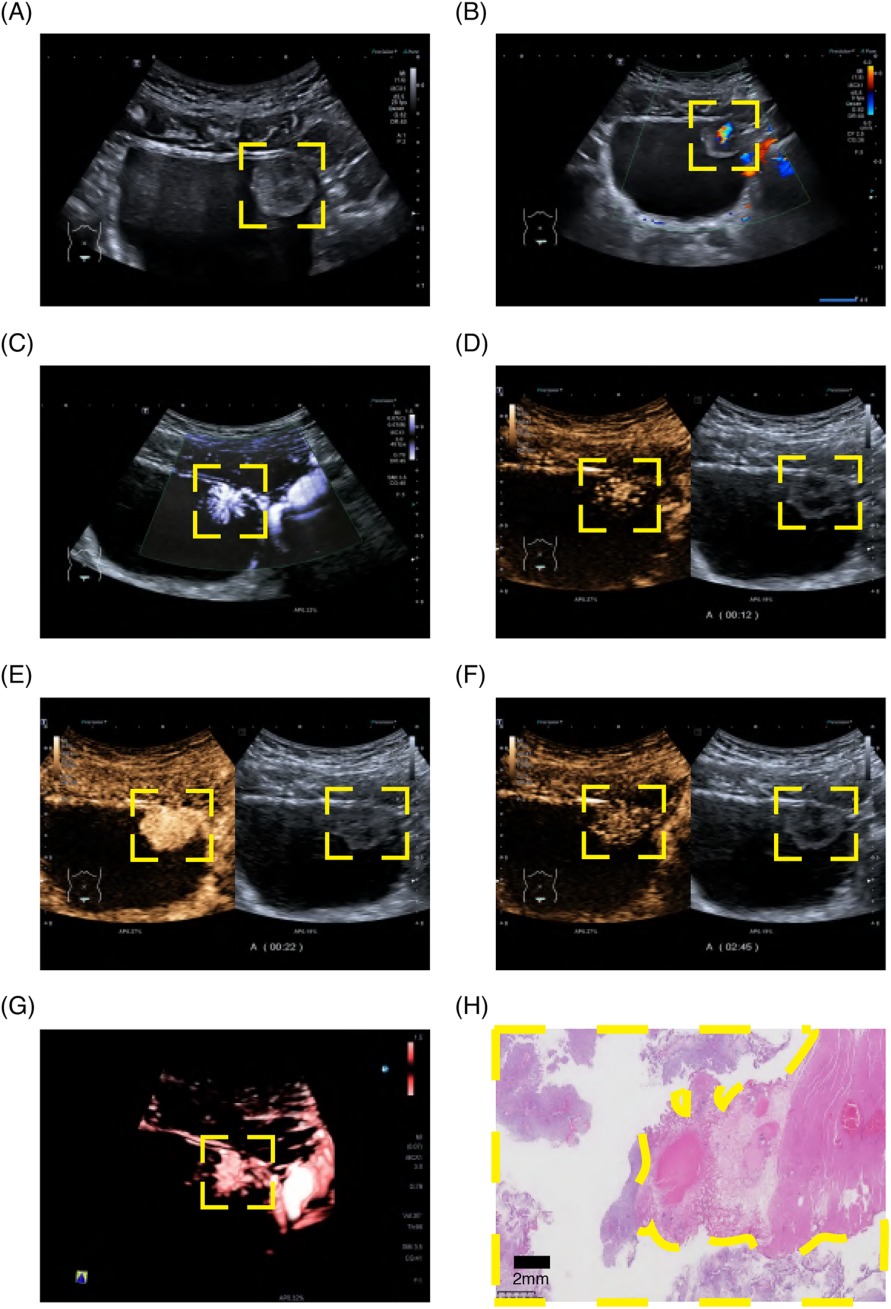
Ultrasound characteristics and pathology status of nonmuscle invasive bladder cancer patients and pathology status. (A) Gray‐scale ultrasound; (B) color Doppler flow imaging; (C) contrast harmonic imaging + superb micro vascular imaging; (D) contrast harmonic imaging in 12 s just before the lesion began to strengthen; (E) contrast harmonic imaging in 22 s almost before the lesion reached the peak; (F) contrast harmonic imaging in 165 s after contrast material was washed out; (G) contrast harmonic imaging + superb micro vascular imaging + smart 3D; (H) pathology imaging. The yellow boxes highlight the lesions.
